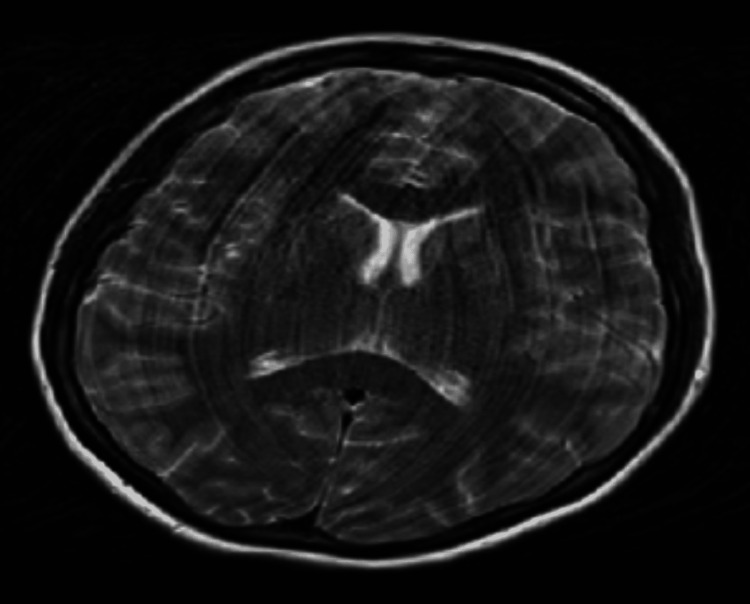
MRI of the brain shows no abnormality
